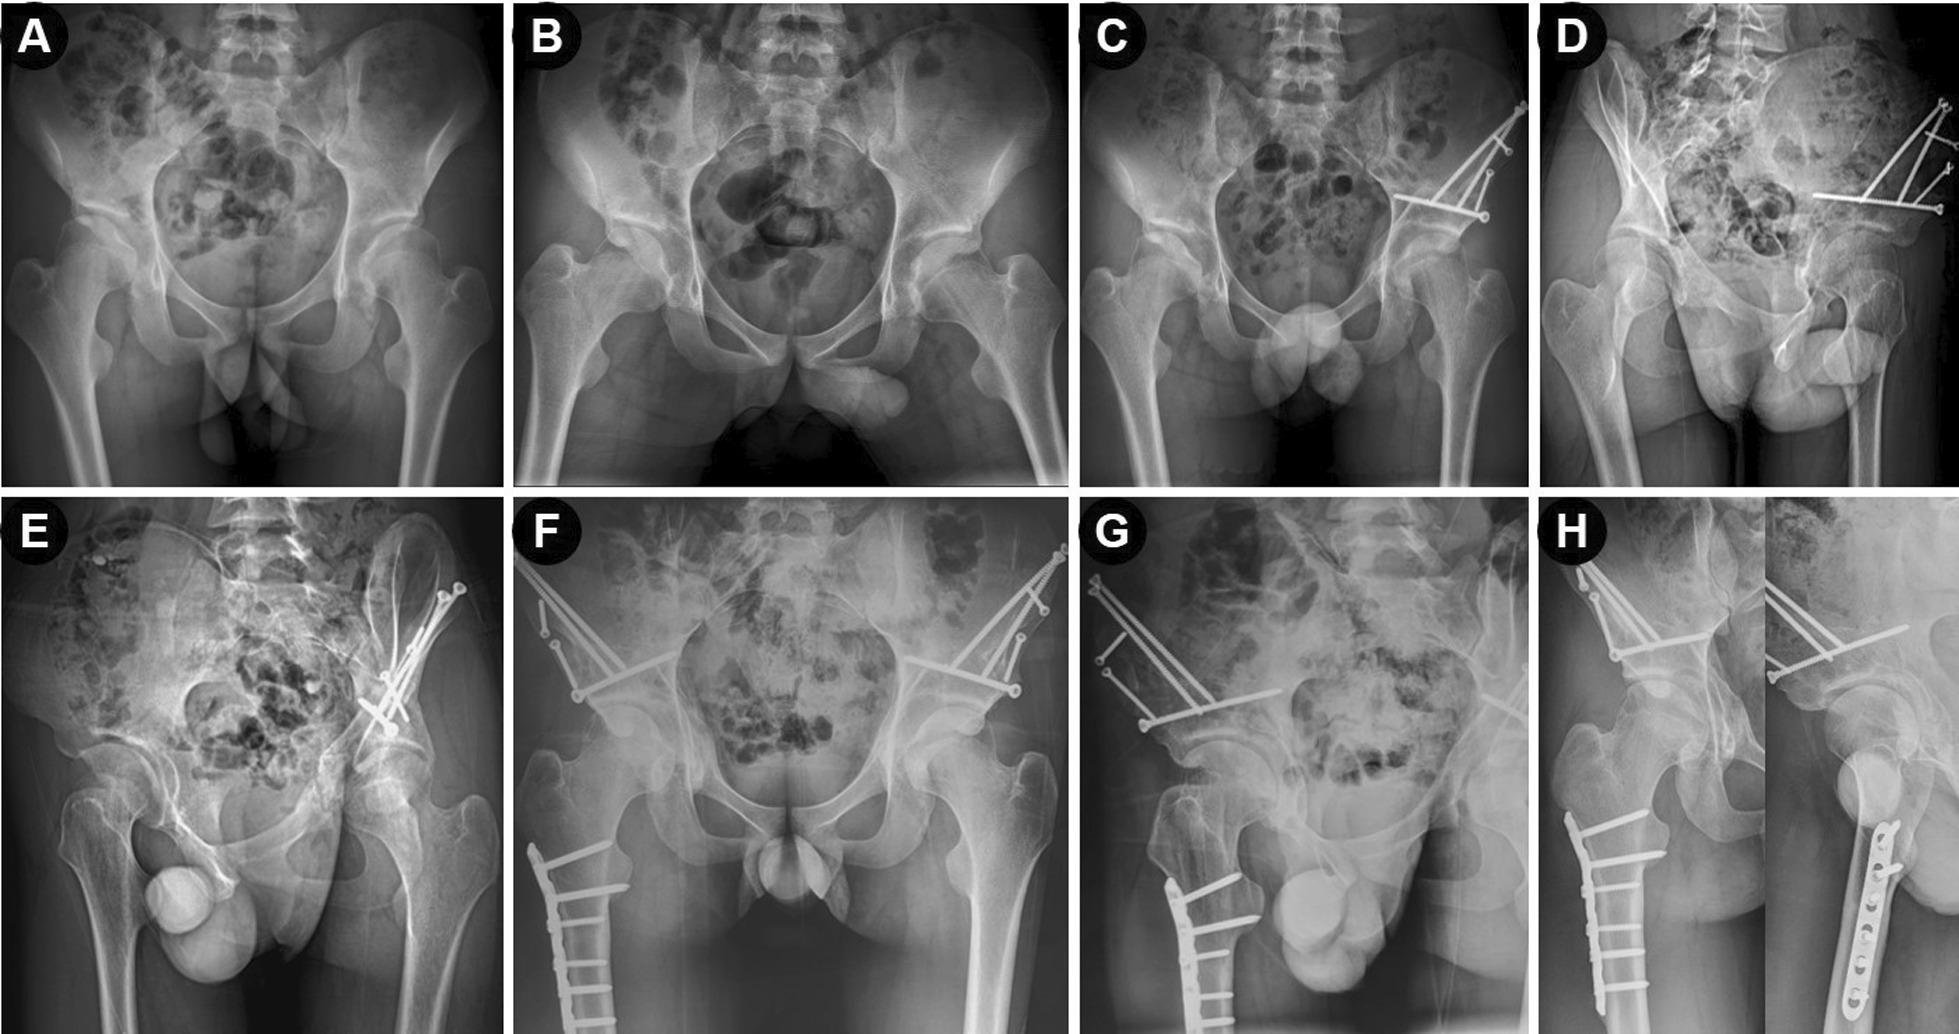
Preoperative radiographs and post-operative correction of an 18 years old man with bilateral hip dysplasia. He was treated by Bernese PAO and femoral derotation osteotomy under the double-incision approach. A, B Preoperative radiographs of the pelvis, showing bilateral acetabular dysplasia. C, D Postoperative anteroposterior pelvic radiograph and false-profile radiograph of the left hip at 3 months. E Preoperative false-profile radiographs of the right hip. F–H Postoperative radiographs at 1 year (left hip) and 9 months (right hip), showing good reconstruction of the hips
